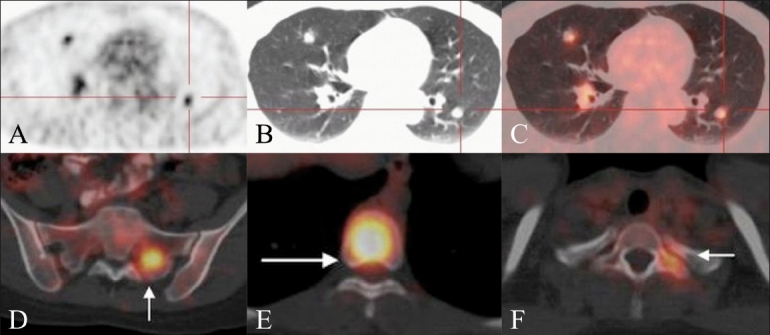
Restaging FDG PET / CT studies performed on two different patients of treated CRC. Axial PET (A), axial CT scan (B), and fused axial PET / CT (C) images show multiple FDG-avid lung metastases. Axial fused PET / CT images of another patient of CRC show FDGavid skeletal metastases in the left sacral ala (arrow in D), D8 vertebral body (arrow in E), and in the left transverse process of the D1 vertebra (arrow in F)
